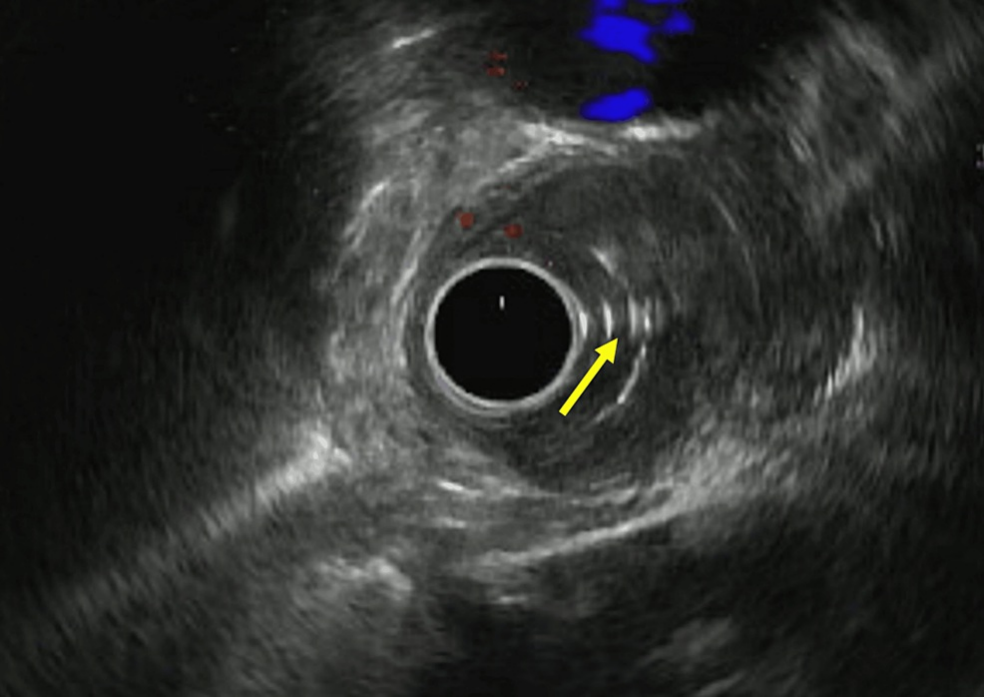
EUS depicting esophageal SCC classified as stage 3: Tumor-3 (T3) and Nodes-2 (N2). EUS: endoscopic ultrasound; SCC: squamous cell carcinoma
